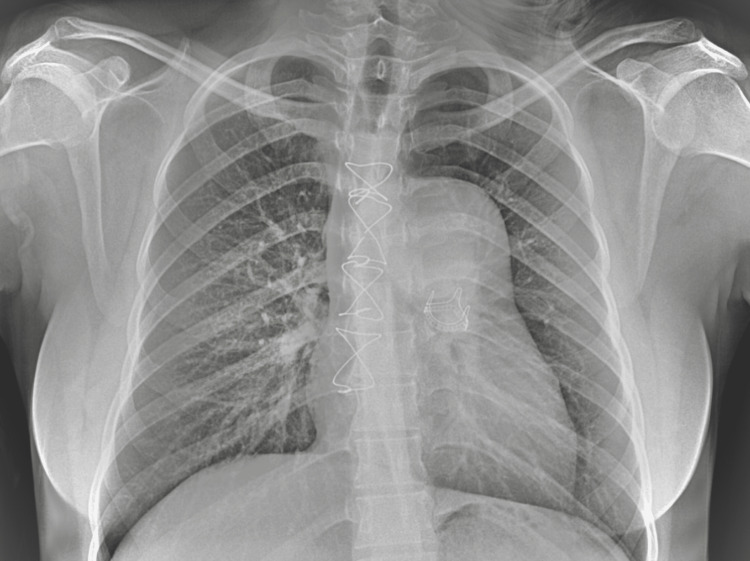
Preoperative X-ray. Chest radiograph obtained prior to surgical intervention showing an implanted 21 mm pulmonary bioprosthesis and aneurysmal dilation of the pulmonary trunk (~30 mm).
